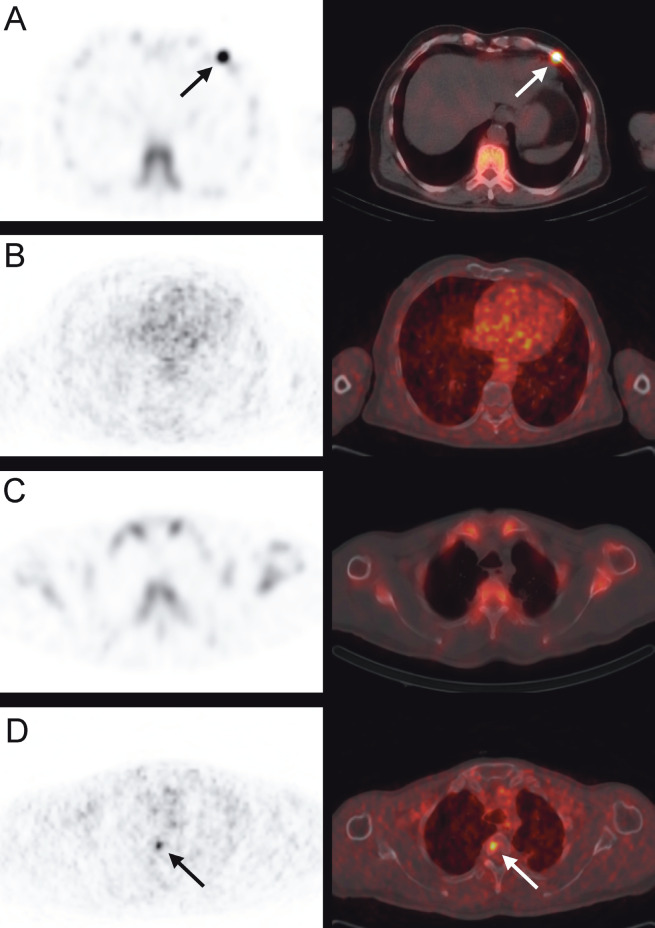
Tc-99m MDP bone scintigraphy SPECT/CT (A and C) and Ga-68 PSMA PET/CT (B and D) images of the same prostate cancer patient. While the rib lesion with increased uptake on bone scan (A) is negative on PSMA PET/CT (B), PSMA PET/CT shows additional uptake on third dorsal vertebra (D) (arrow), which is negative on bone scan (C).
